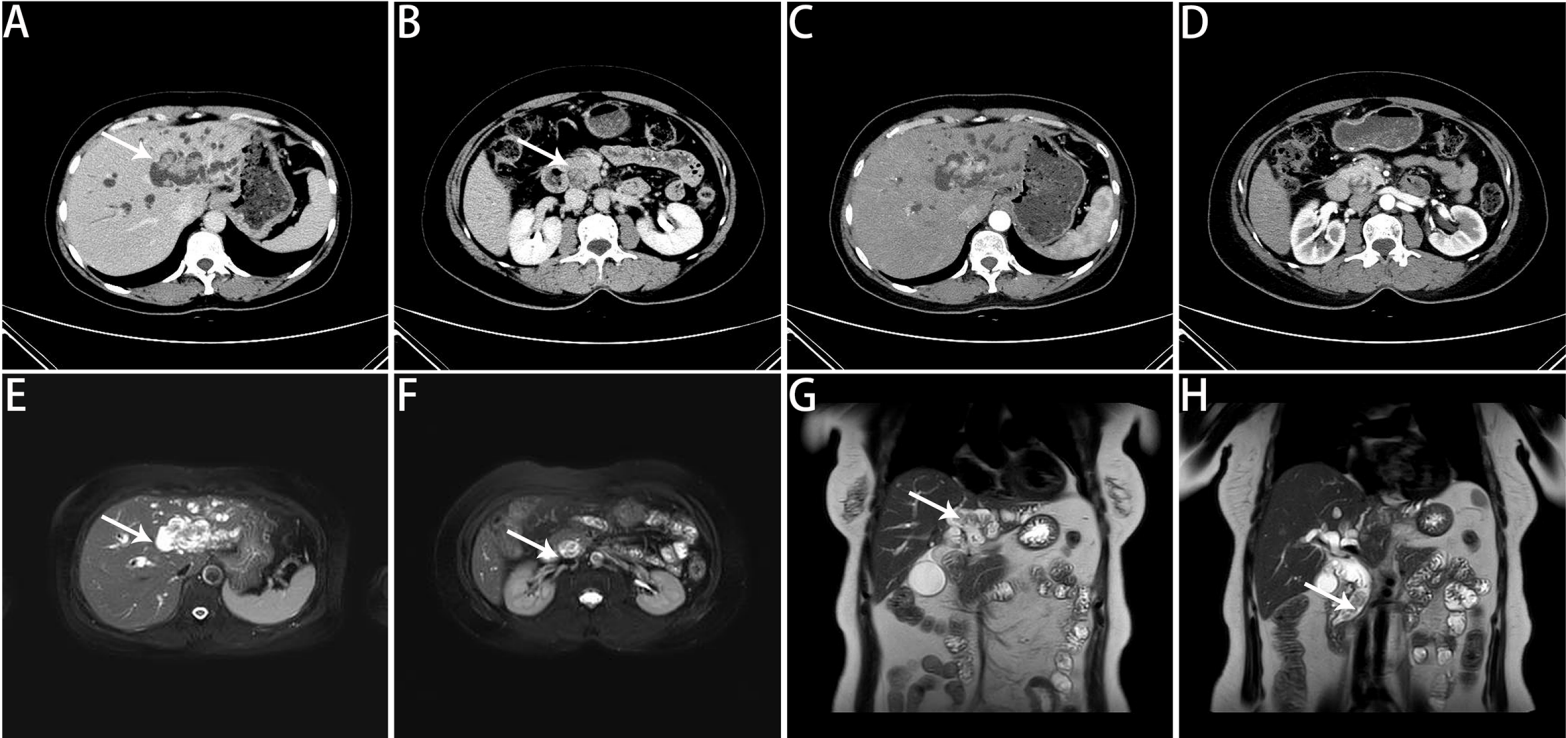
Preoperative imaging findings. (A) Noncontrast CT scan revealing multiple isodense nodules within the left intrahepatic bile ducts. (B) Noncontrast CT scan showing multiple isodense nodules within the distal common bile duct. (C) CE-CT in the arterial phase revealing space-occupying lesions with marked enhancement and irregular borders in the left intrahepatic bile ducts. (D) CE-CT in the arterial phase revealing markedly enhanced space-occupying lesions in the distal common bile duct. (E) MRCP image revealing multiple irregular space-occupying lesions in the left intrahepatic bile ducts. (F) MRCP image showing multiple irregular space-occupying lesions in the distal common bile duct. (G) MRCP coronal view showing multiple irregular space-occupying lesions in the left intrahepatic bile ducts. (H) MRCP coronal view showing multiple irregular space-occupying lesions in the distal common bile duct. The white arrows indicate lesions.
